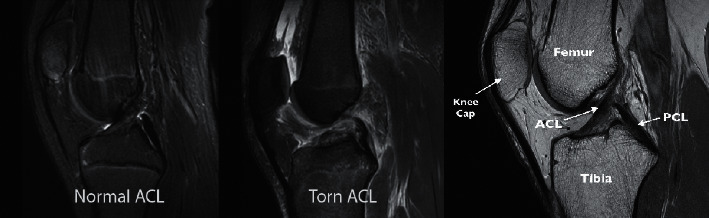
Difference between normal and torn ACL in MRI.
